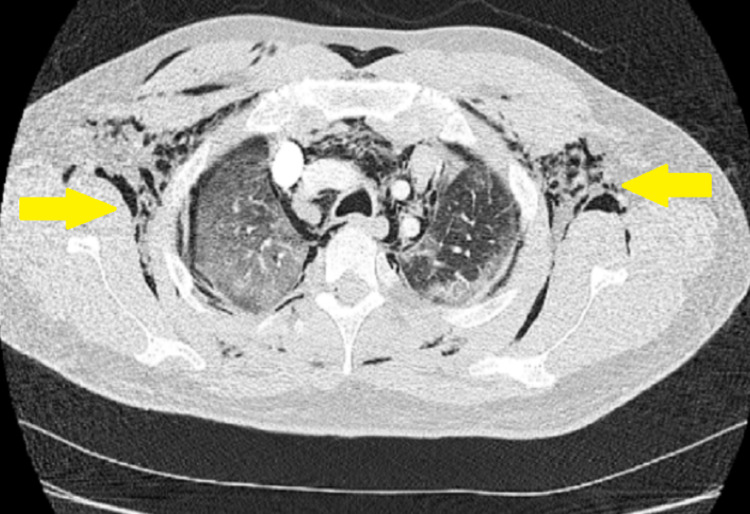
Chest CT axial section of pulmonary parenchymal window showing subcutaneous emphysema (yellow arrows) and extensive ground-glass opacities in the lung parenchyma.
